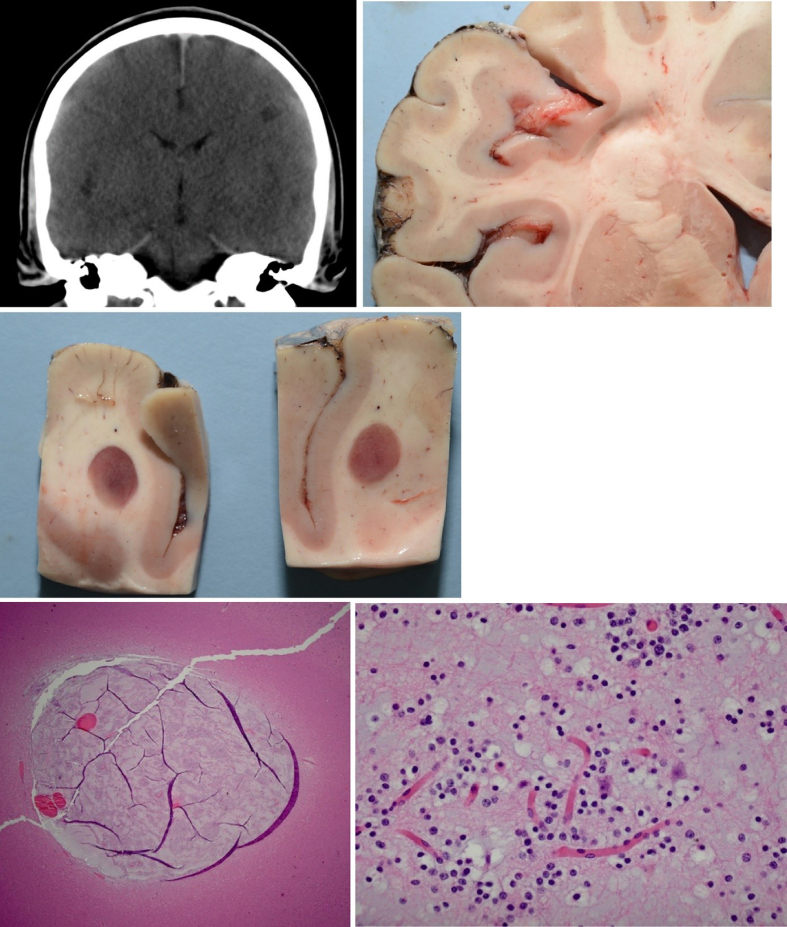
Targeted sampling of radiographic lesions according to Iles [59]. Dysembryoplastic neuroepithelial tumor, CNS
